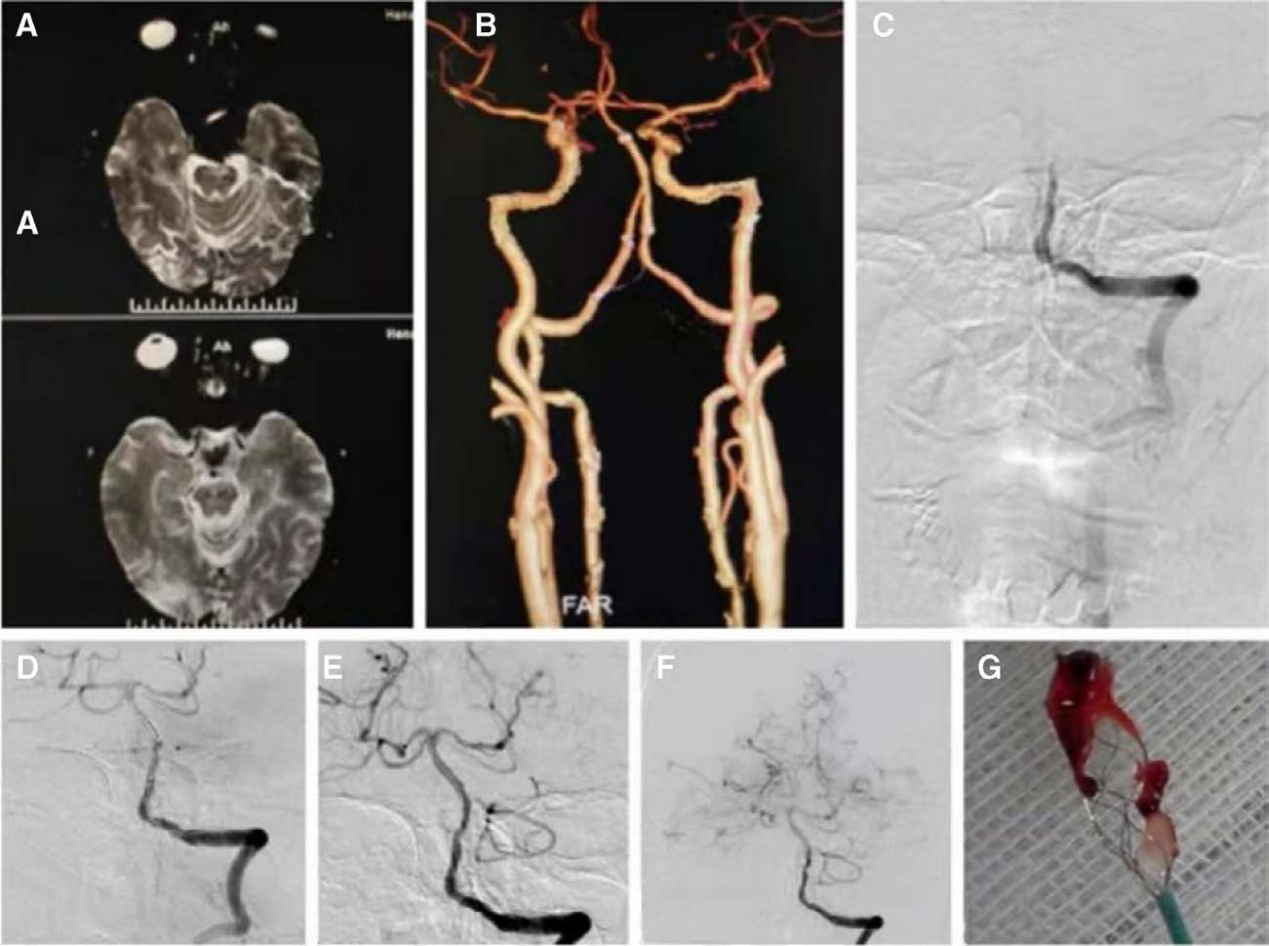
(A) DWI showing ischemic lesions in the left cerebral peduncles. (B) CTA showing basilar artery stenosis. (C) Basilar artery occlusion was confirmed by angiography. (D) The stent was sent through vessel occlusion. (E. F) DSA showed basilar artery blood recovered TICI-3 flow. (G) Thrombosis removed from a stent. CTA = computed tomography angiography.
